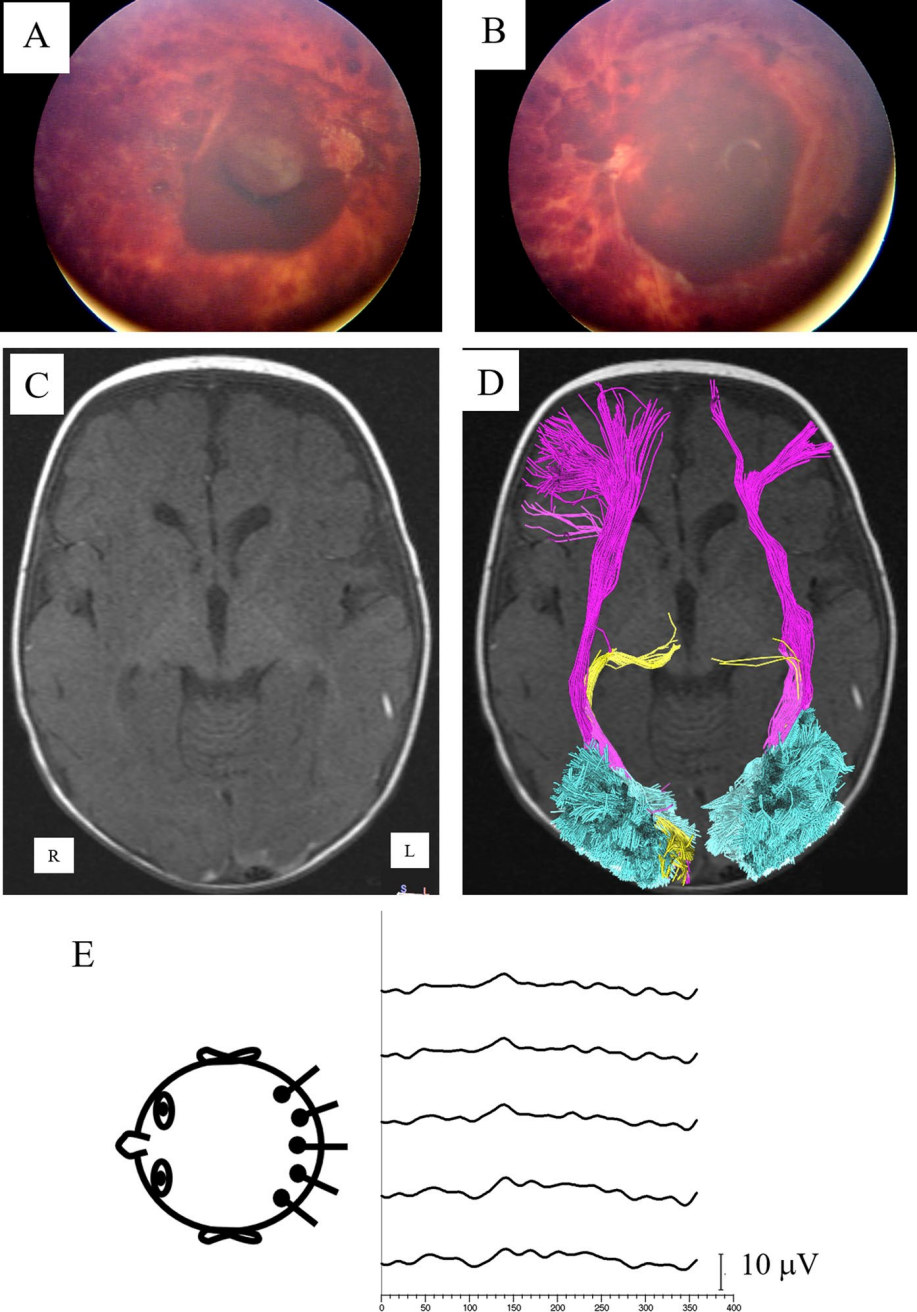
A child presenting at 133 days age with unresponsiveness, seizure-like episodes, subdural hematomas, skull fracture, and leg fractures. RetCam imaging of the right eye A and left eye B show numerous intraretinal, preretinal, subretinal hemorrhages. Both eyes had a traumatic schisis cavity with associated circumferential folds. Both optic discs were swollen. An MRI at 136 days age showed diffuse abnormal FLAIR signal, restricted diffusion within both cerebral and cerebellar hemispheres. C Axial T1-weighted image, D same image with reconstructed tracts superimposed showing reduced volume of the left optic radiations (yellow), intact inferior fronto-occipital fasciculi (purple), and intact volume loss of the vertical occipital fasciculi. (cyan). E, VEP at 0.7 years age showed significantly reduced responses of delayed latency. At last follow-up visual acuity was 0.86 logMAR and he had global developmental delay and autism disorder
